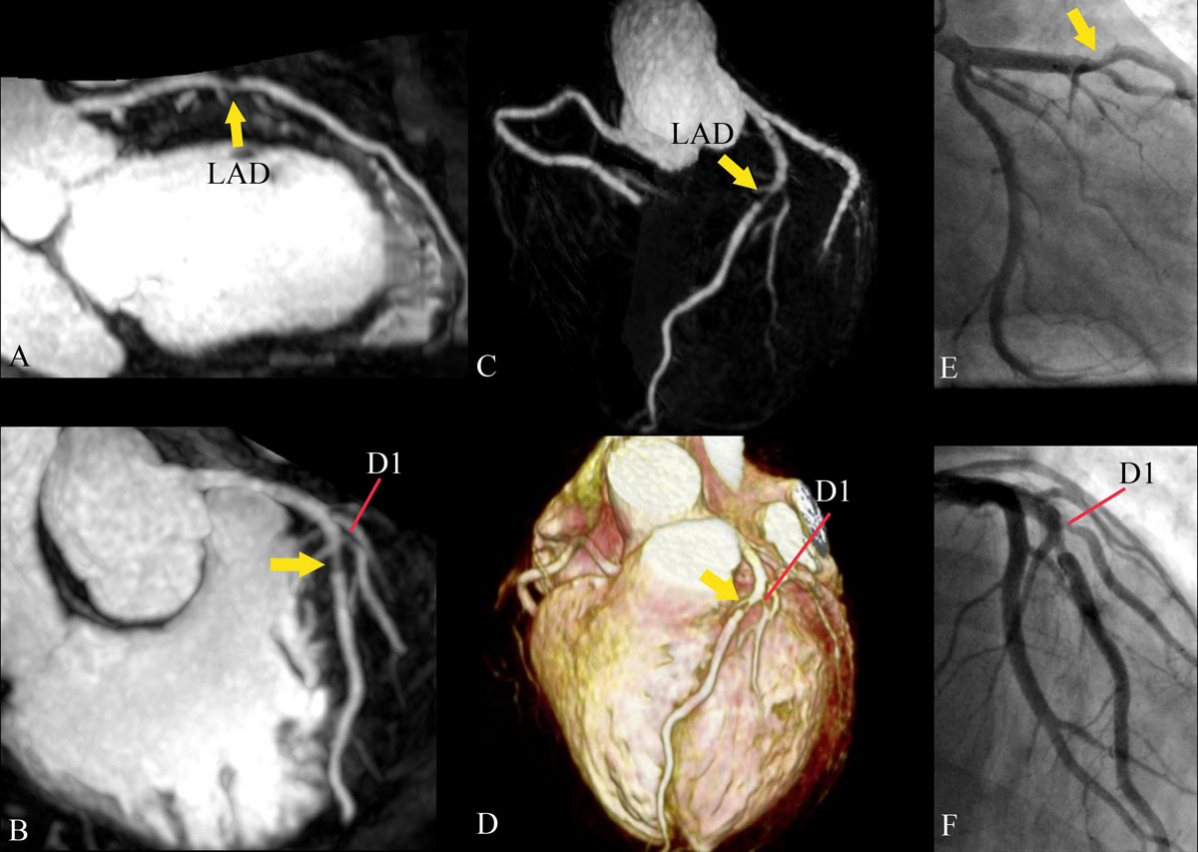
Curved planar reconstruction (CPR) image (A), Sliding thin slab maximum intensity projection (MIP) image (B), MIP image of coronary tree (C), and volume-rendered image (D) detect coronary artery stenoses in the LAD (arrow) and first diagonal branch (arrowhead). Good agreement is observed between coronary MRA and X-ray coronary angiography.
